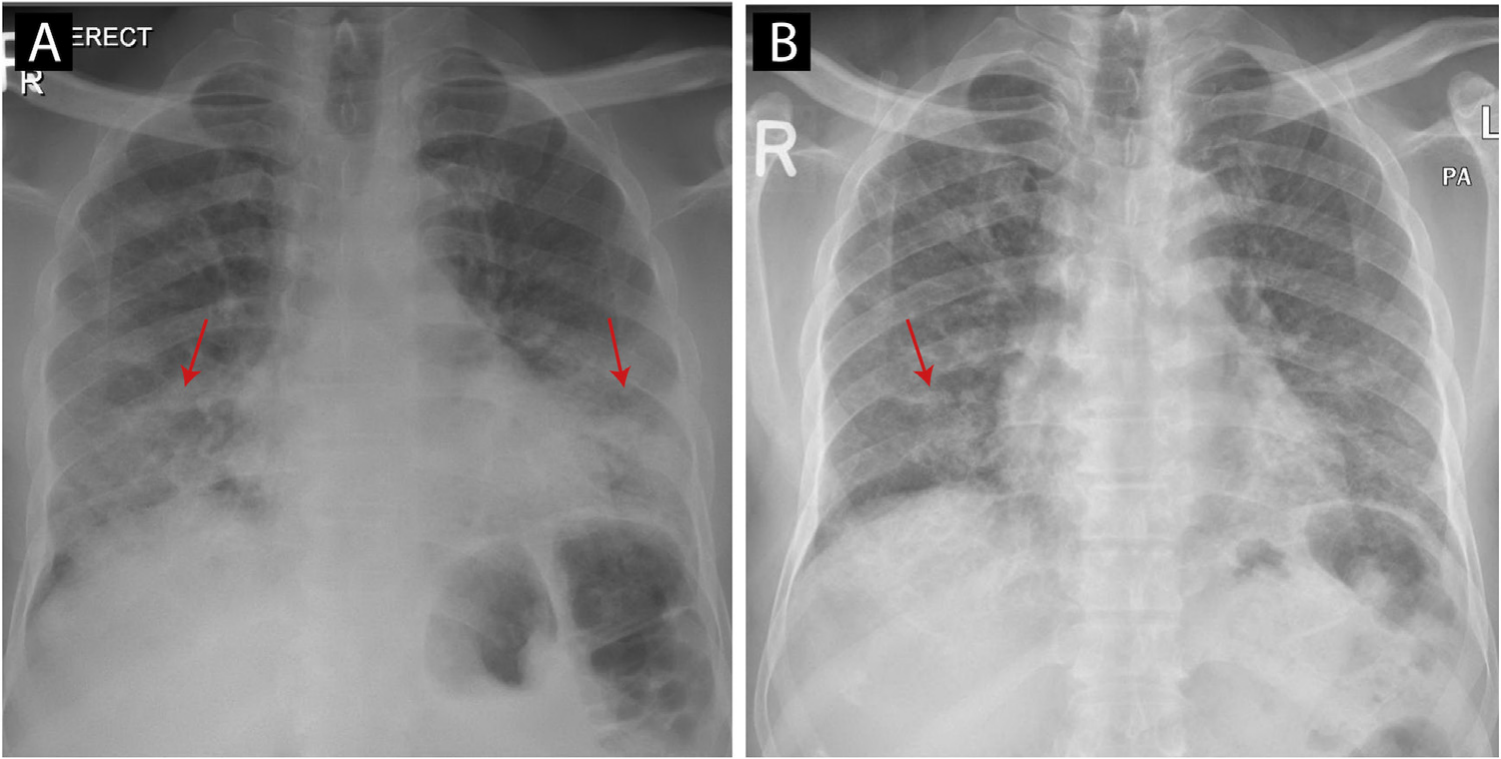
Chest Xray (1a: at the time of COVID-19 infection, discrete areas of airspace consolidation in the bilateral lower and mid zones. 1b: on the second admission, showing resolution of the airspace consolidations in the bilateral lower and mid zones).
